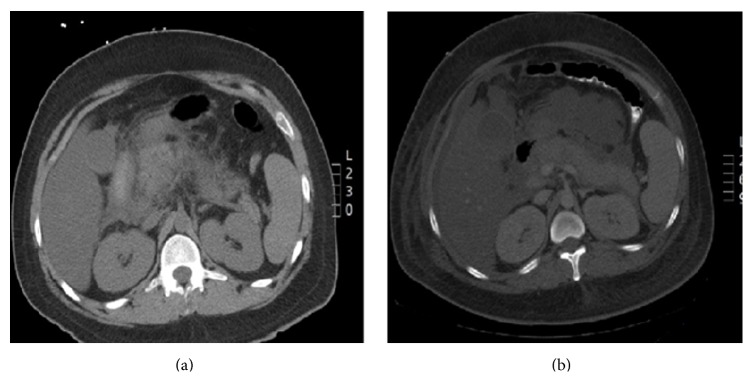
(a) Initial abdomen CT: enlarged pancreas with peripancreatic inflammatory changes consistent with pancreatitis. (b) Interval worsening of the acute pancreatitis. More extensive retroperitoneal fluid extending anteriorly along the psoas muscles more on the right side down to the deep pelvis. Hypoechoic areas in the head of the pancreas/uncinate process possibly areas, perfusion deficits, and parenchymal necrosis. Fluid in the peripancreatic region, lesser sac, and small free intraperitoneal fluid more on the right abdomen.
